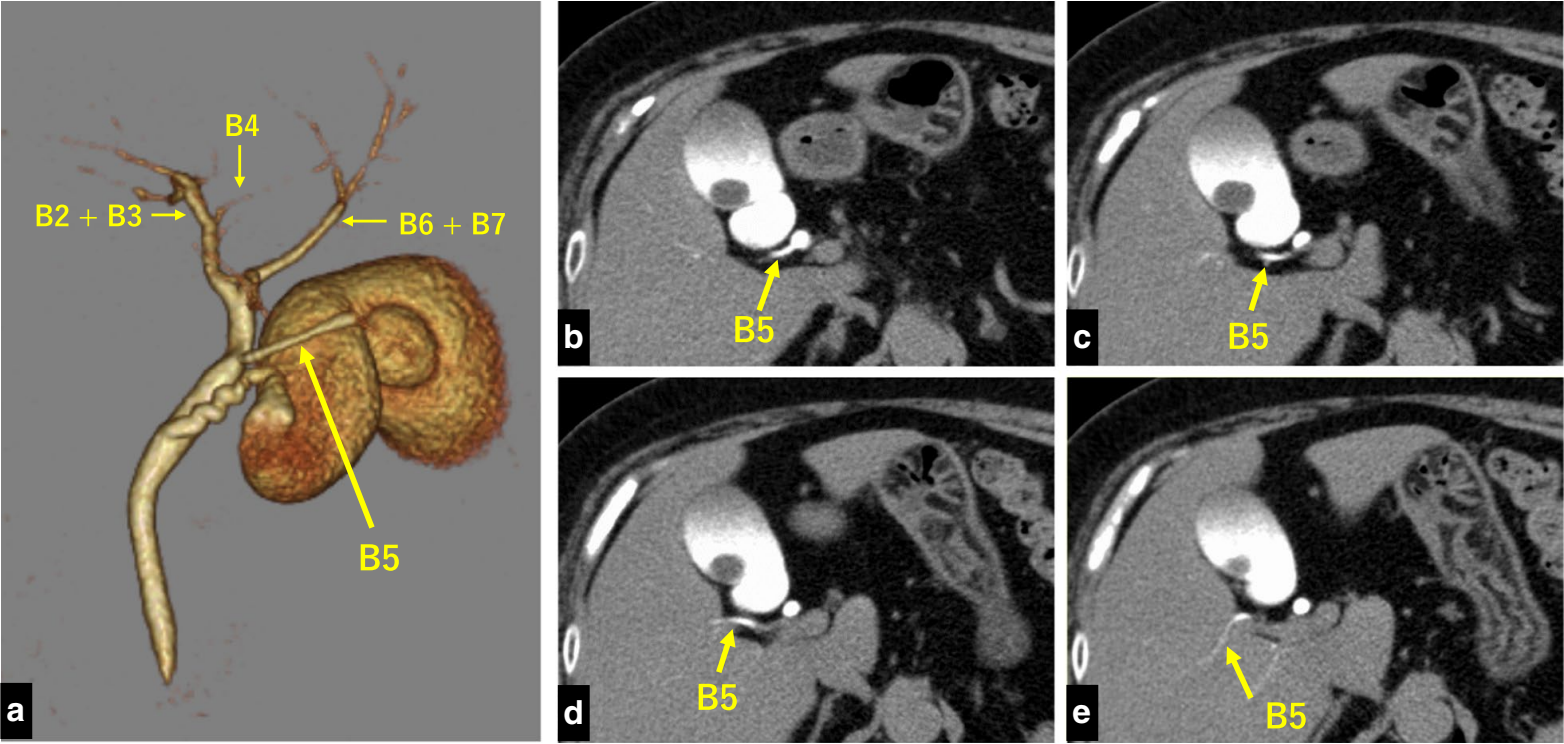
A 69-year-old woman with cholelithiasis and aberrant biliary duct draining segment 5. Drip-infusion cholangiography-computed tomography accurately demonstrating the aberrant biliary duct of segment 5 branching directly off the common hepatic duct near the confluent section of the cystic duct and the common hepatic duct
